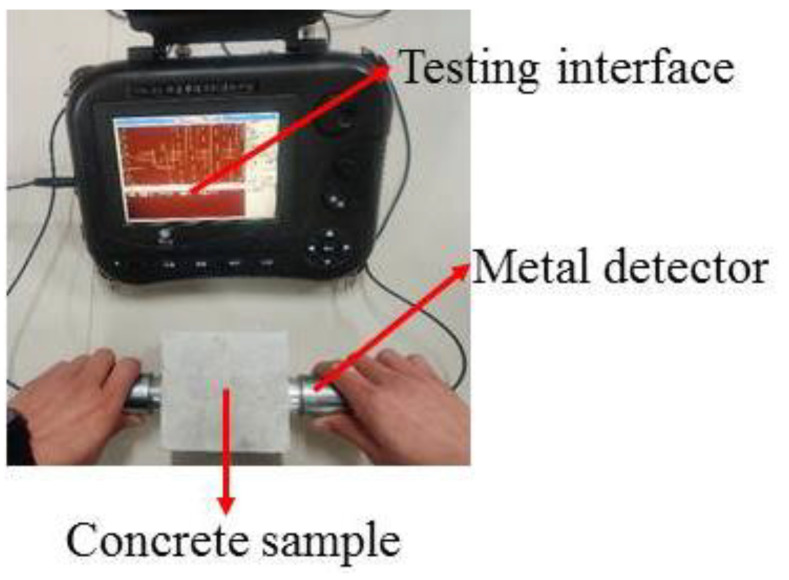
NA-M4 nonmetal ultrasonic detector.
